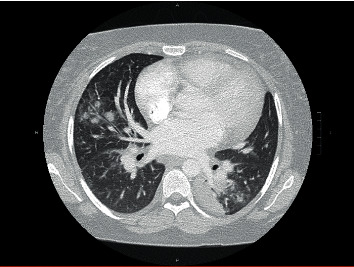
Computed tomography pulmonary angiogram showing no pulmonary embolism; multifocal pulmonary infiltrates; small right-sided pleural effusion; very small pericardial effusion; cardiomegaly.
